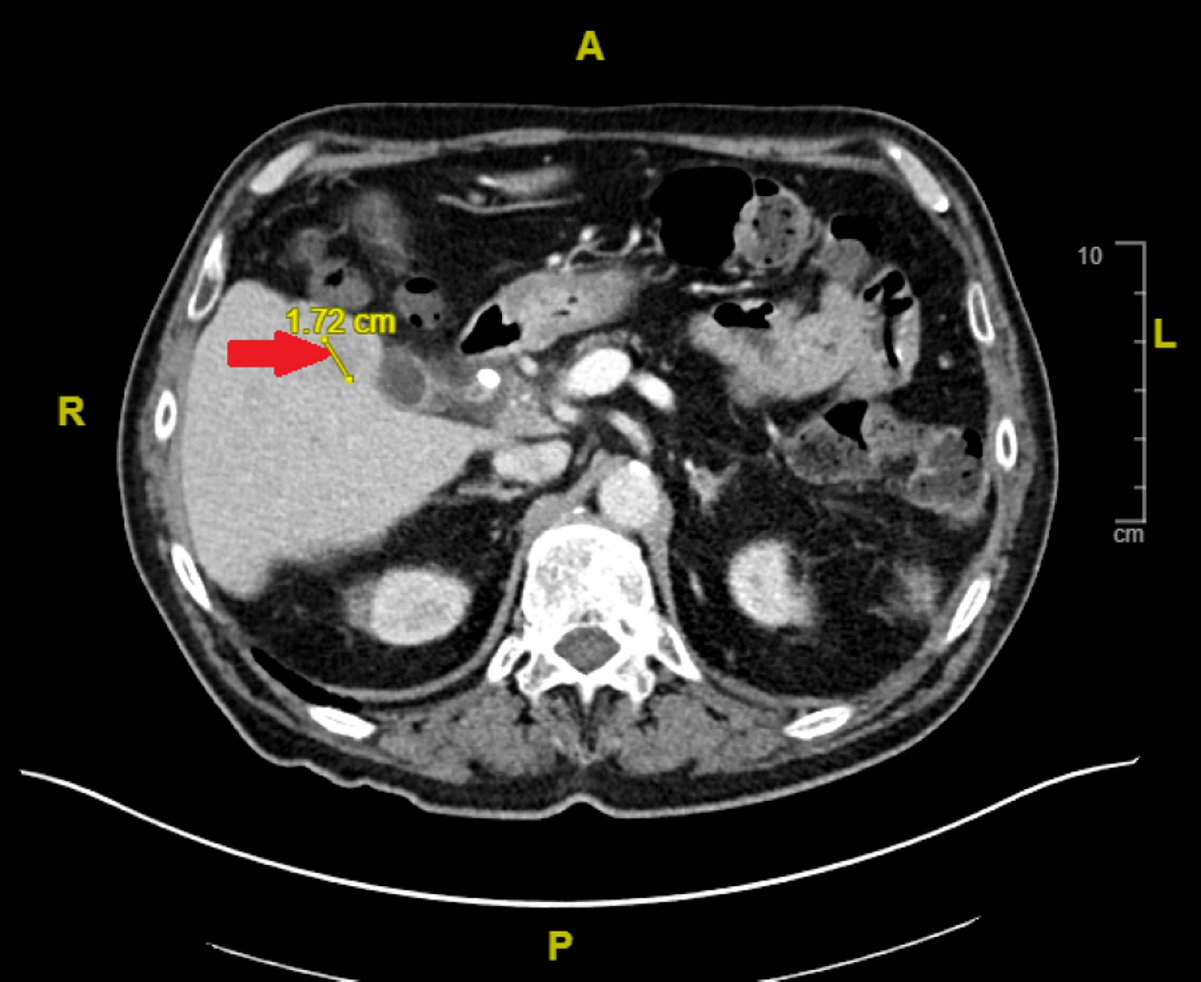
Cross-sectional computed tomography (CT) scan of abdomen and pelvis with intravenous contrast Red arrow: Since the CT from 2.5 weeks ago, there is near resolution of the segment V multilocular lesion adjacent to the gallbladder and interval decrease in the larger inferior segment V multilocular lesion, with no fluid components visualized on the current CT. The slightly low-density residual lesion is approximately 1.8 cm
